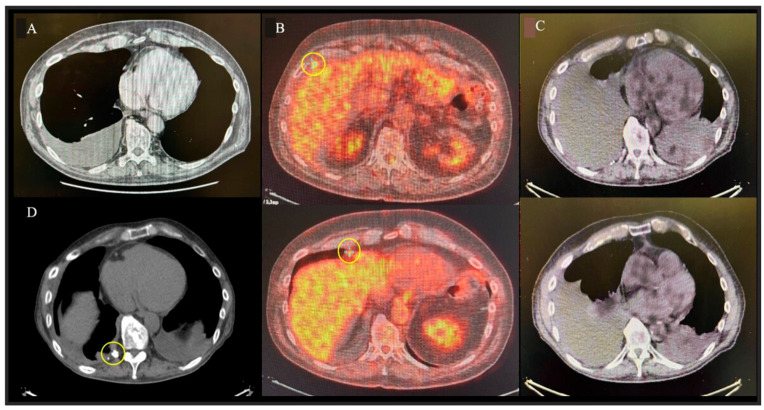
Imaging findings. (A): Chest CT before first PITAC showing right pleural effusion. (B): PET-CT before first PITAC showing two FDG-avid right pleural lesions (circled). (C): PET-CT before second PITAC showing effusion recurrence without FDG uptake. (D): Follow-up CT one year after second PITAC showing no effusion recurrence; biopsy site clips are circled.
